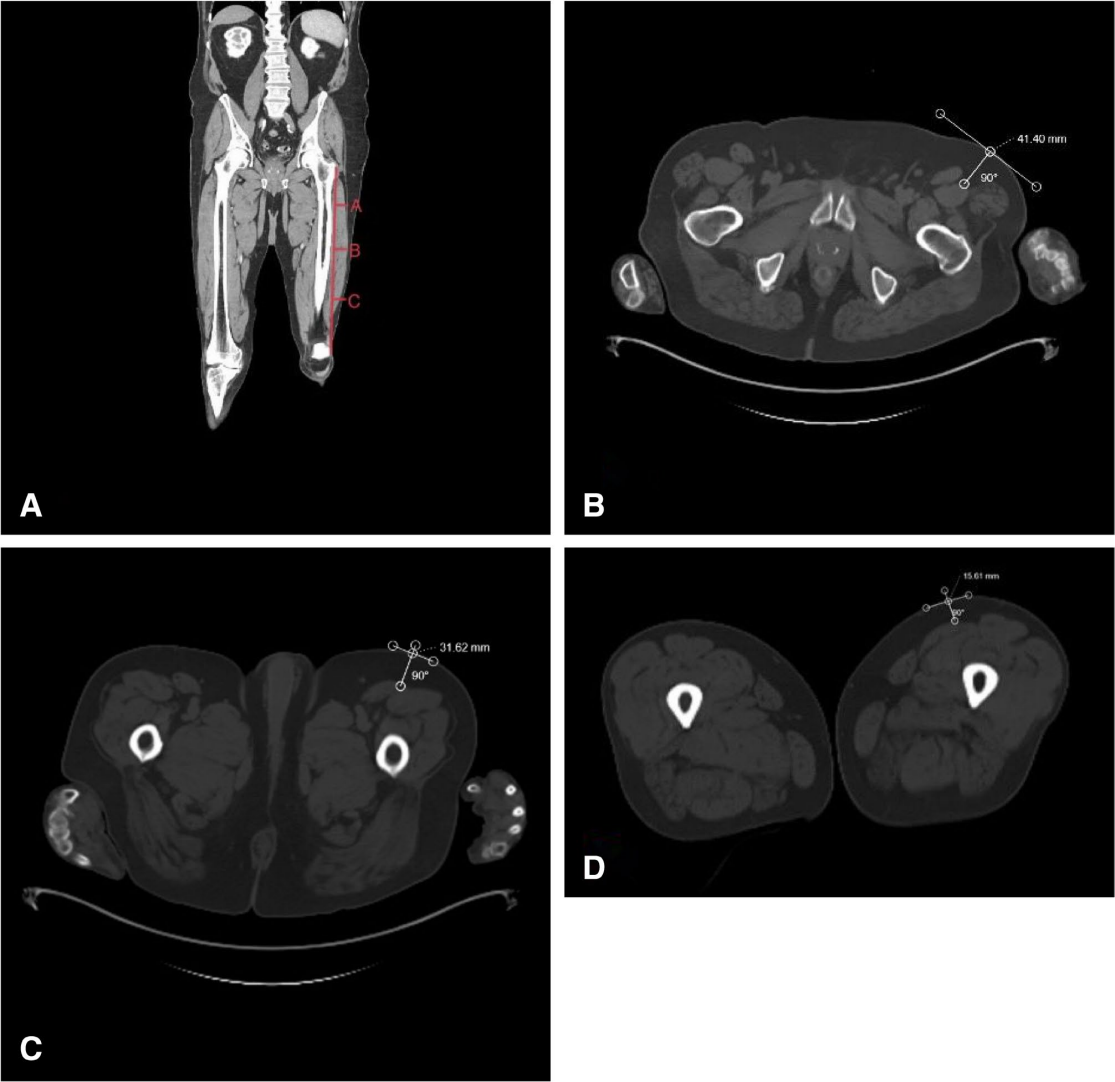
Measurement of ALTFF thickness along the thigh using a CT scan to guide harvest planning. (A) Line connecting the anterior superior iliac spine (ASIS) to the superior lateral surface of the patella. Thickness measurements were obtained at three points: (B) Point A, located at the proximal fourth of the line, (C) Point B, at the midpoint, and (D) Point C, the distal fourth. [Color figure can be viewed in the online issue, which is available at www.laryngoscope.com]
